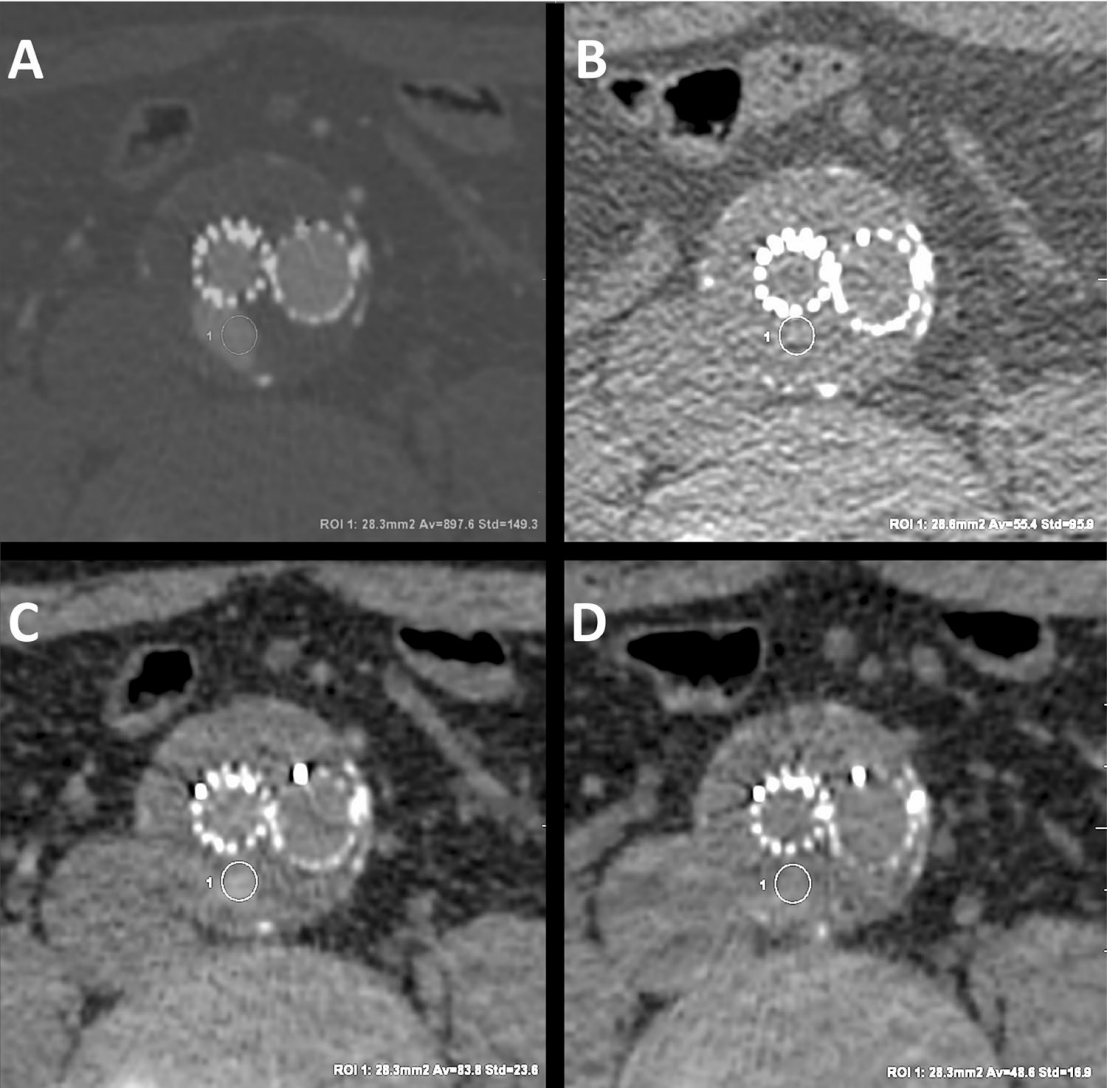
Images presenting differences in CT numbers (average ± SD) in the region of type II endoleak in arterial, true non-contrast (TNC) and 2 virtual non-contrast (VNC) phases. Reconstructions: 40 keV VMI arterial phase (A—897 ± 149 HU), TNC (B—55 ± 95 HU), VNCa (C—83 ± 23 HU), VNCd (D—48 ± 16 HU). Same axial slice, same patient. TNC, VNCa, VNCd images presented with the same window settings (W 350, L 40)
